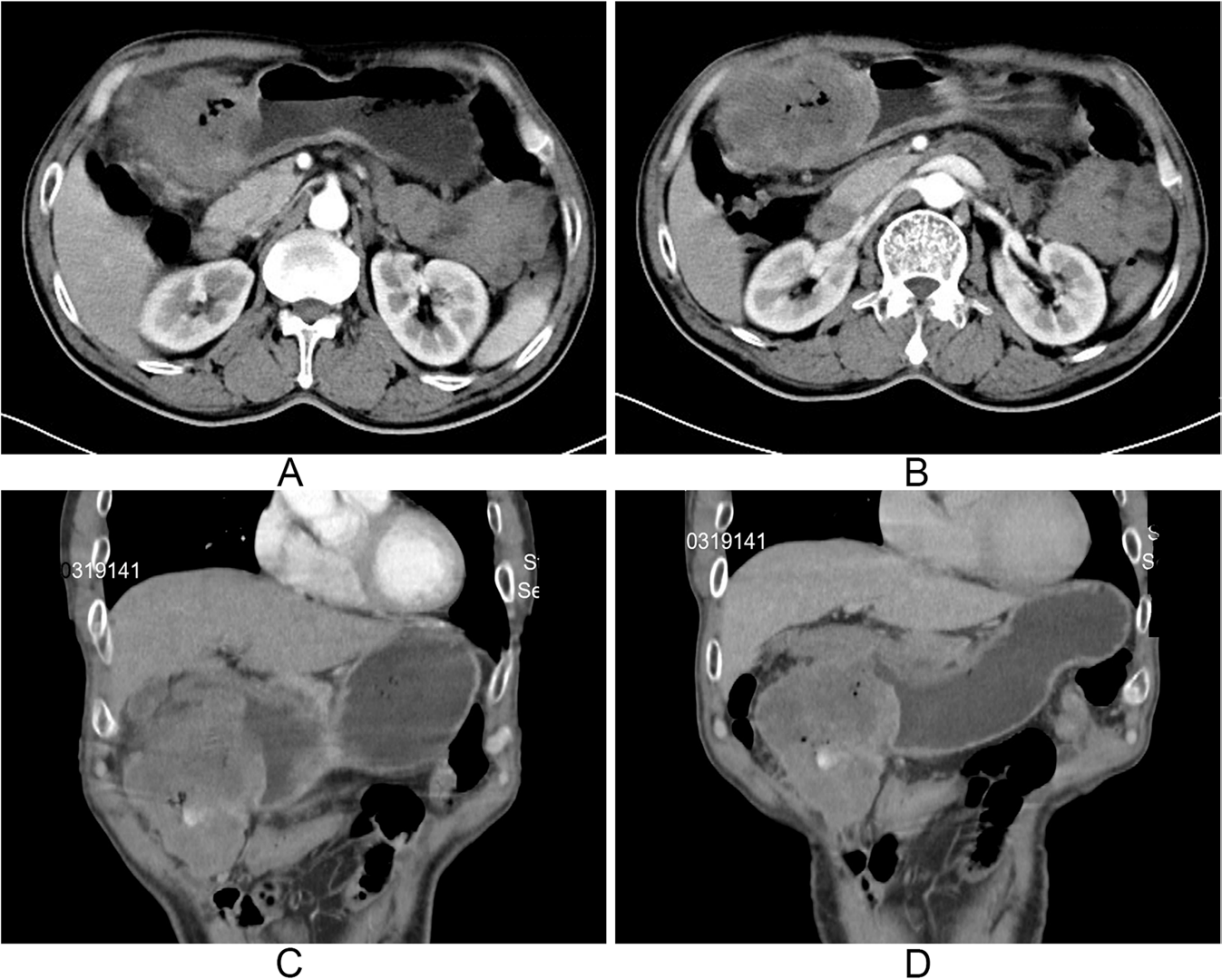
Enhanced abdominal CT scan indicated a abdominal mass with mild peripheral enhancement. The mass seemed to connect to the gastric antrum. A, B The cross-sectional images and C, D the sagittal images
